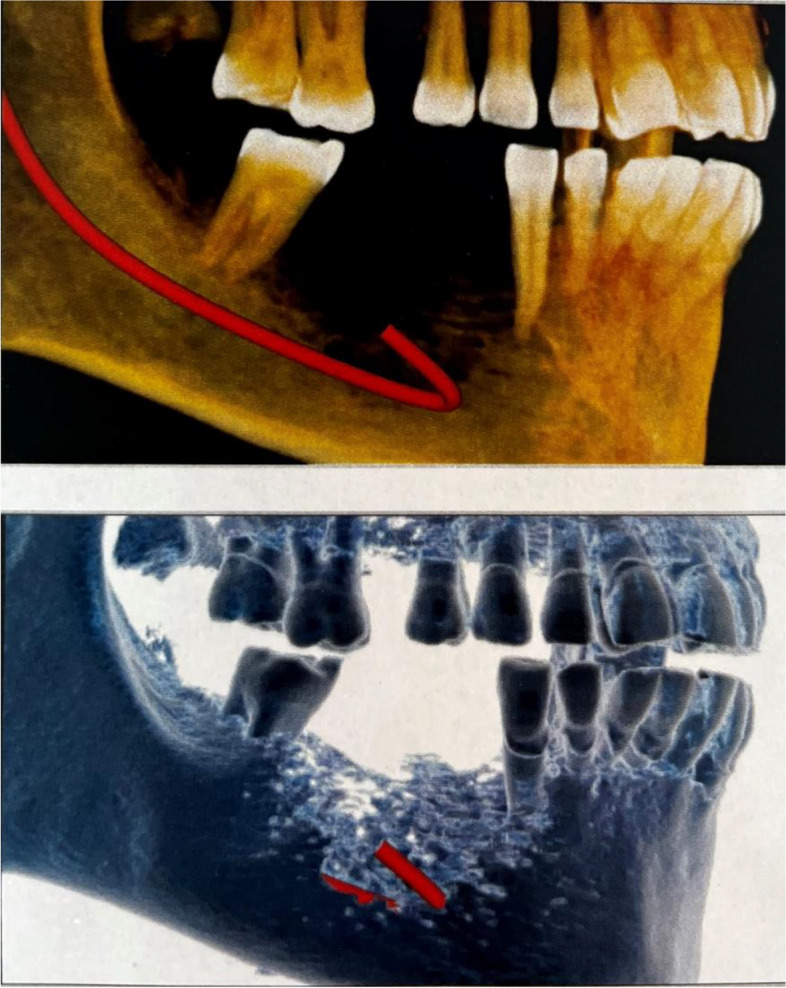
A CBCT image showing large radiolucent lesion related to the lower right molar area with inferior alveolar nerve involvement. B Negative image showing trabecular bone infiltration by tumor cells
